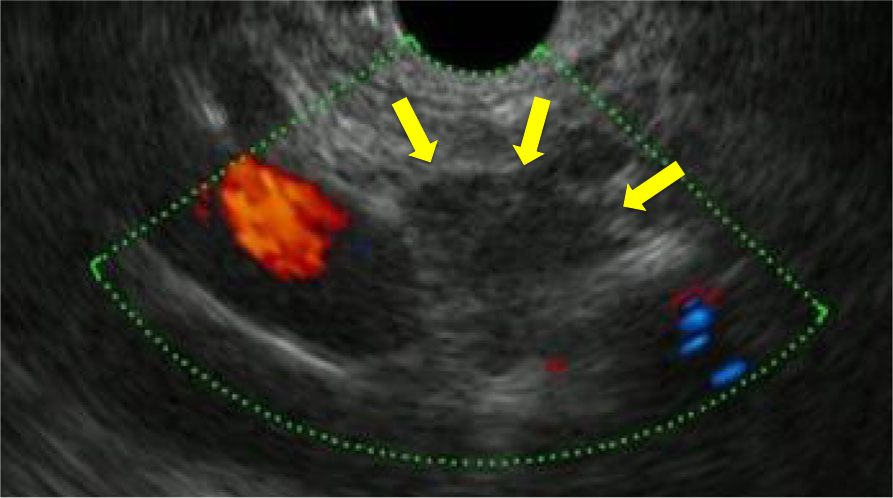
Endoscopic ultrasonography image of the nodule. An endoscopic ultrasonography shows a hypoechoic lesion without vascular flow. The margin of the lesion was slightly indistinct (arrows)
